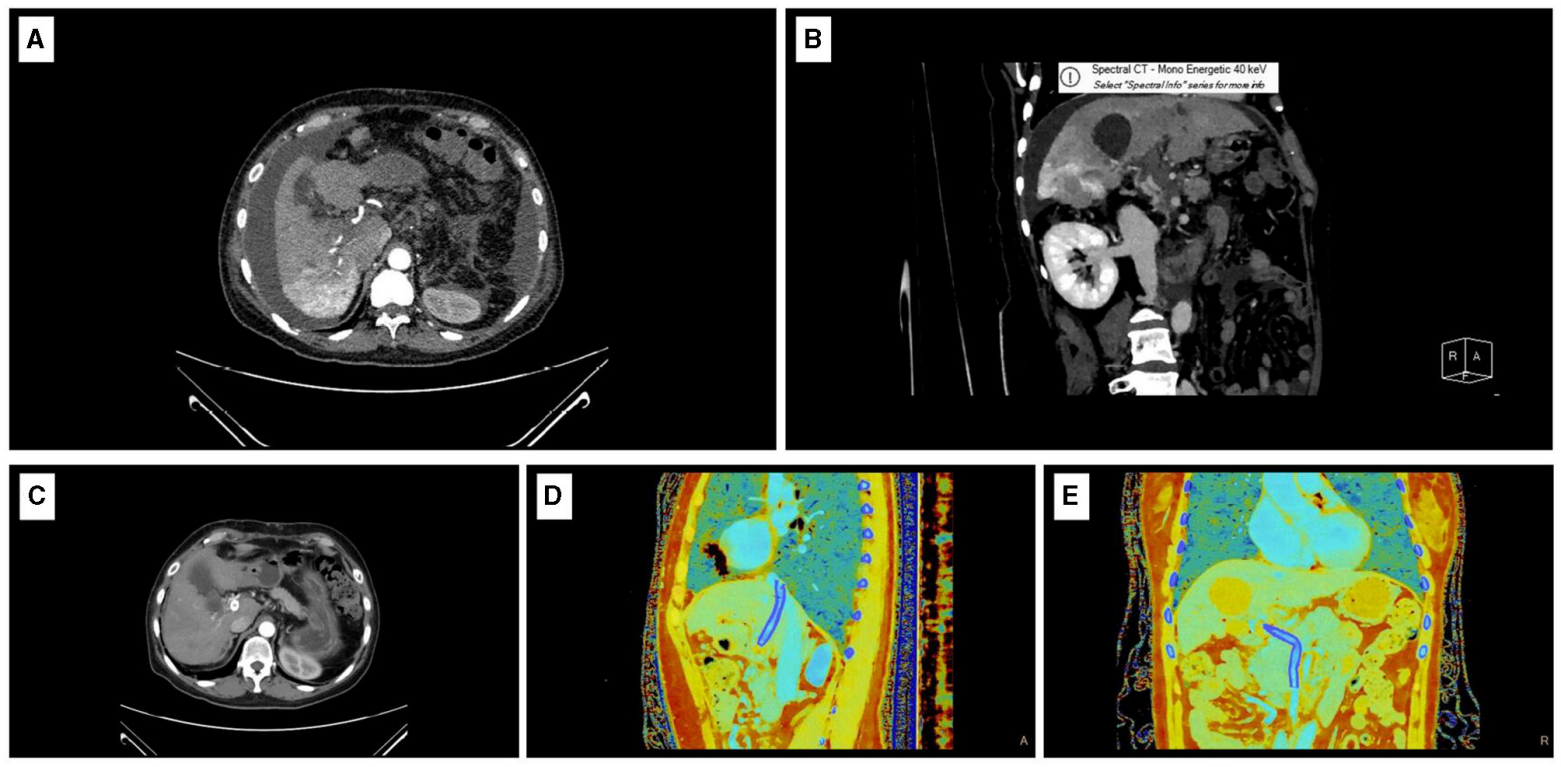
Imaging data. (A) Preoperative CT-enhanced arterial phase transverse image. (B) Preoperative CT-enhanced portal venous phase coronal image. (C) Postoperative CT-enhanced arterial phase transverse image. (D) Sagittal effective atomic number map on postoperative CT. (E) Coronal effective atomic number map on postoperative CT.
